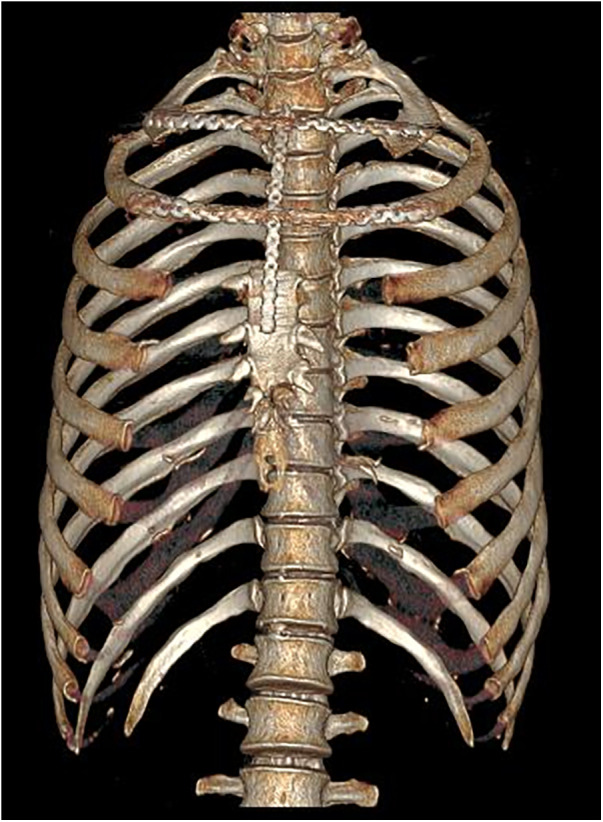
Postoperative three-dimensional reconstruction CT.
